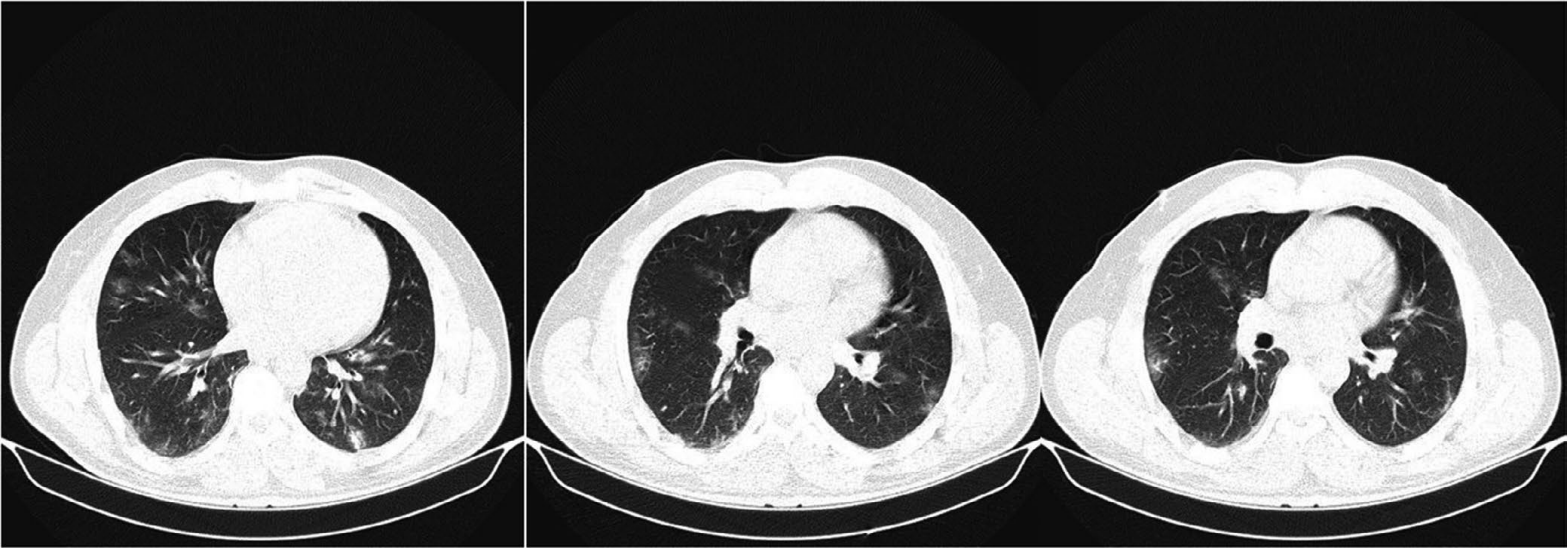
Chest computed tomography with diffuse ground glass hyperdensities involving both lungs. Some non‐significant mediastinal lymph nodes are also detected. These findings are suspicious for COVID‐19
